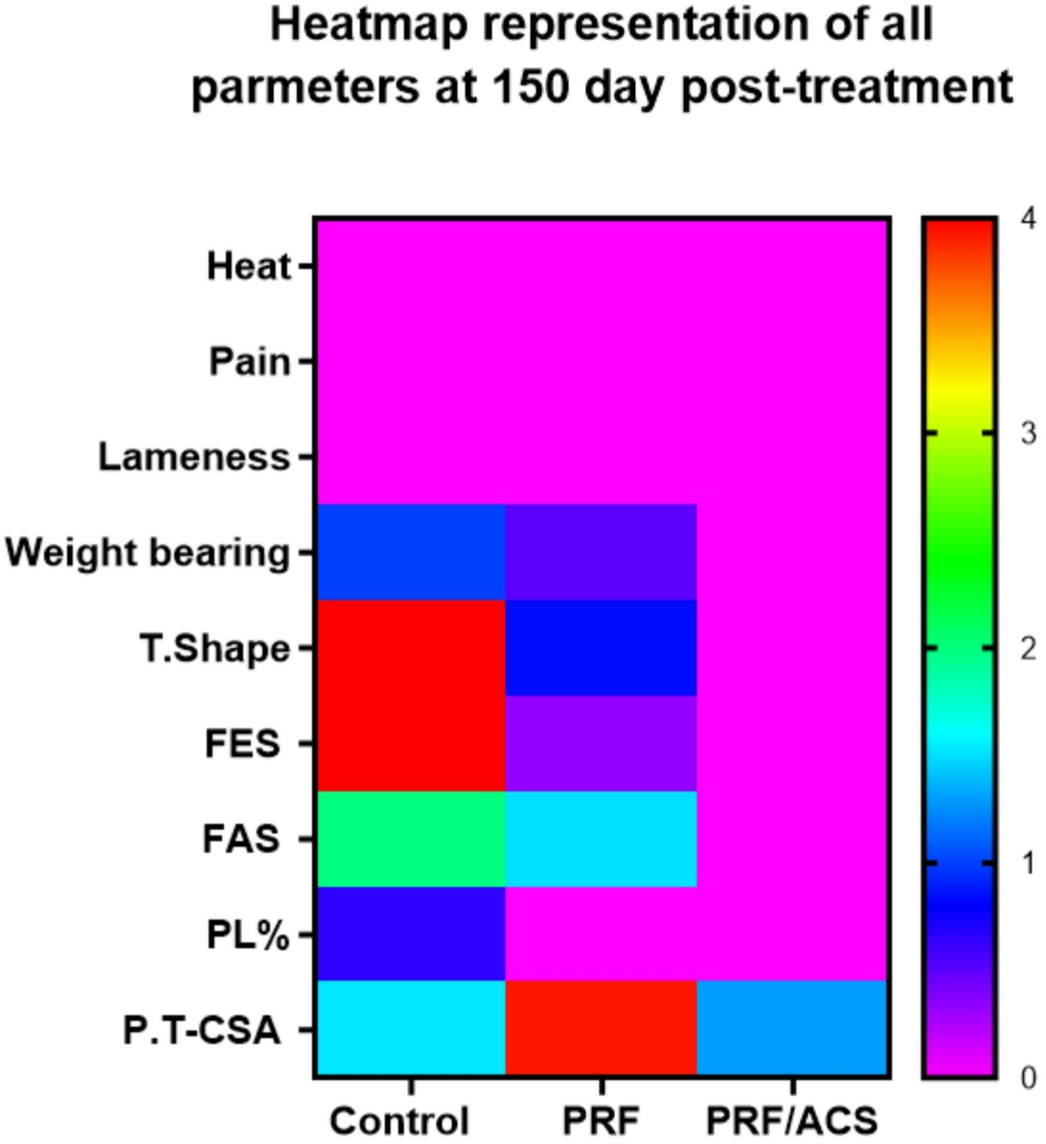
Heatmap reppresentation of all clinical and ultrasongraphical evaluation parameters in all groups at the end of the study (T150)
